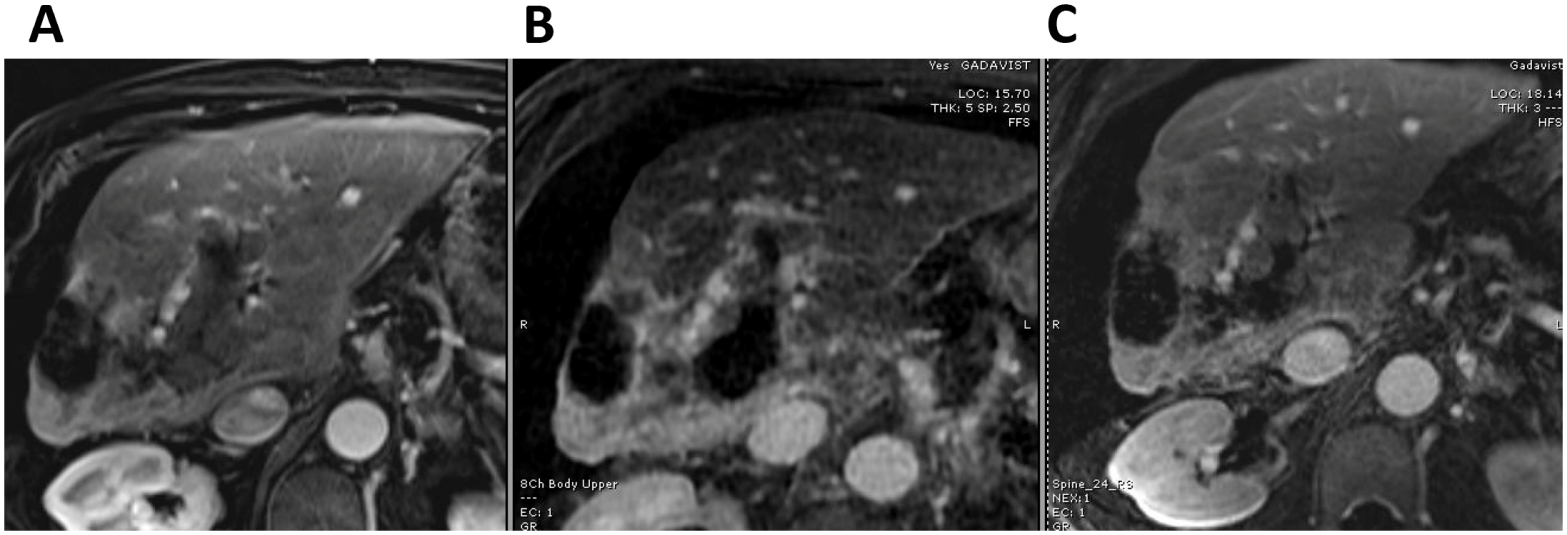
Axial contrast-enhanced T1-weighted MR images of the liver showing the treatment effect of histotripsy in the portal vein tumor thrombus prior to histotripsy (A) and six-weeks (B) and four months (C) post histotripsy showing the degree of cavitation of the portal vein tumor thrombus due to histotripsy.
